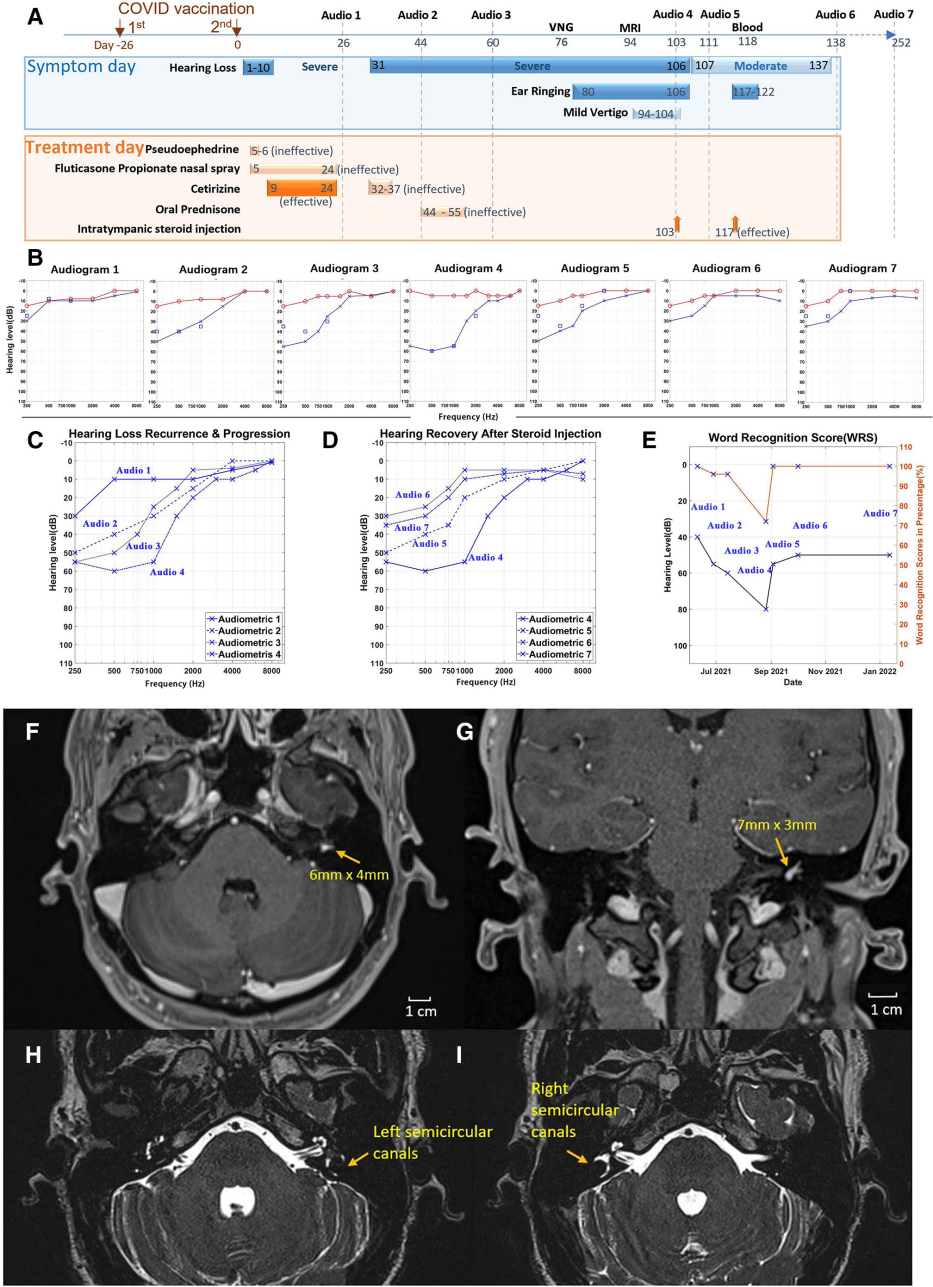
The timeline of clinical symptoms and treatments, audiometric and hearing measurement data, and MRI images of intralabyrinthine schwannoma. (A) The timeline for the two-dose COVID-19 vaccination (4 weeks apart); emerging symptoms of the first 10-day hearing loss starting on day 1 after the second dose of the BNT162b2 mRNA vaccine (day 0), the second (recurrent) hearing loss on day 31 lasting for 3.5 months along with ear ringing and mild vertigo; clinical tests (7 audiometric tests, MRI and blood draw), and the ineffective and effective treatments, including pseudoephedrine (ineffective), fluticasone propionate nasal spray (ineffective), first effective and second ineffective cetirizine treatments, ineffective oral prednisone, and final effective intratympanic steroid injections. No pure tone average abnormality or hearing loss symptoms were reported before vaccination. (B) Standard pure tone audiograms of seven sequential hearting tests at the indicated times in (A) for both right ear (red line) and left ear (blue line). Red circle represents air conduction. Blue square represents bone conduction and blue cross represents air conduction. (C) The accumulative audiogram of four left ear audiometric tests (1–4) showing the second hearing loss progression over 2 months. (D) The accumulative audiogram of four left ear audiometric tests (4–7) showing hearing recovery and stability after local steroid treatments to the left ear. (E) Left ear hearing level (air conduction) and word recognition score (WRS) in percentage (%) at the longitudinal series of 7 tests before and after intratympanic steroid injections. (F–I) Gadolinium-enhanced MRI of axial contrast-enhanced (F) and sagittal contrast-enhanced (G) volumetric T1w images with an intralabyrinthine schwannoma (arrow-pointed) in the left ear, and axial contrast-enhanced volumetric T2w images with the left semicircular canals (blurry) (H) and with the right semicircular canals (clear) (I).
